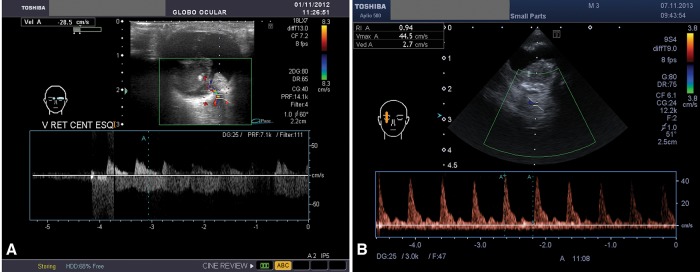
Color Doppler image coupled with a 16 MHz ultrasonography image of a unilateral retinoblastoma. The central retinal artery and central retinal vein were identified together in the middle of the optic nerve ultrasonography image and were then assessed from the posterior scleral surface (A) up to approximately 10 mm behind this landmark (B). The flow above the x-axis shows a peaked wave and represents the central retinal artery; the wave below this axis is more undulated and represents the central retinal vein. The spot assessed is denoted by the two parallel lines crossed by an oblique line to indicate the Doppler angle, which is ideally between 30° and 60°. The blue cross and blue ‘A’ were added manually to the wave to measure the velocity, which may be quite high in the central retinal artery of tumor-containing eyes (B).
